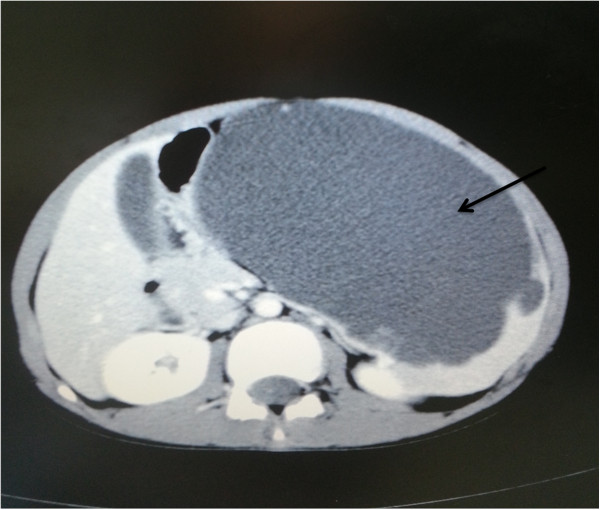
Computed tomography scans suggestive of a hypodense cystic lesion arising from the spleen.
